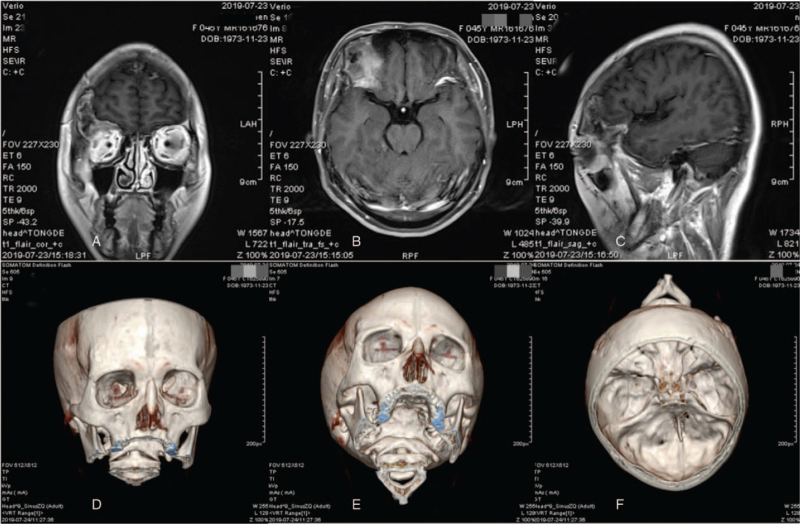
Neoplastic lesion in the right frontal/supraorbital and anterior skull base regions in case 2: different phases of enhancing magnetic resonance imaging (A–C) and three-dimensional reconstruction of the skull tumor by high-resolution computer tomography (D–F).
